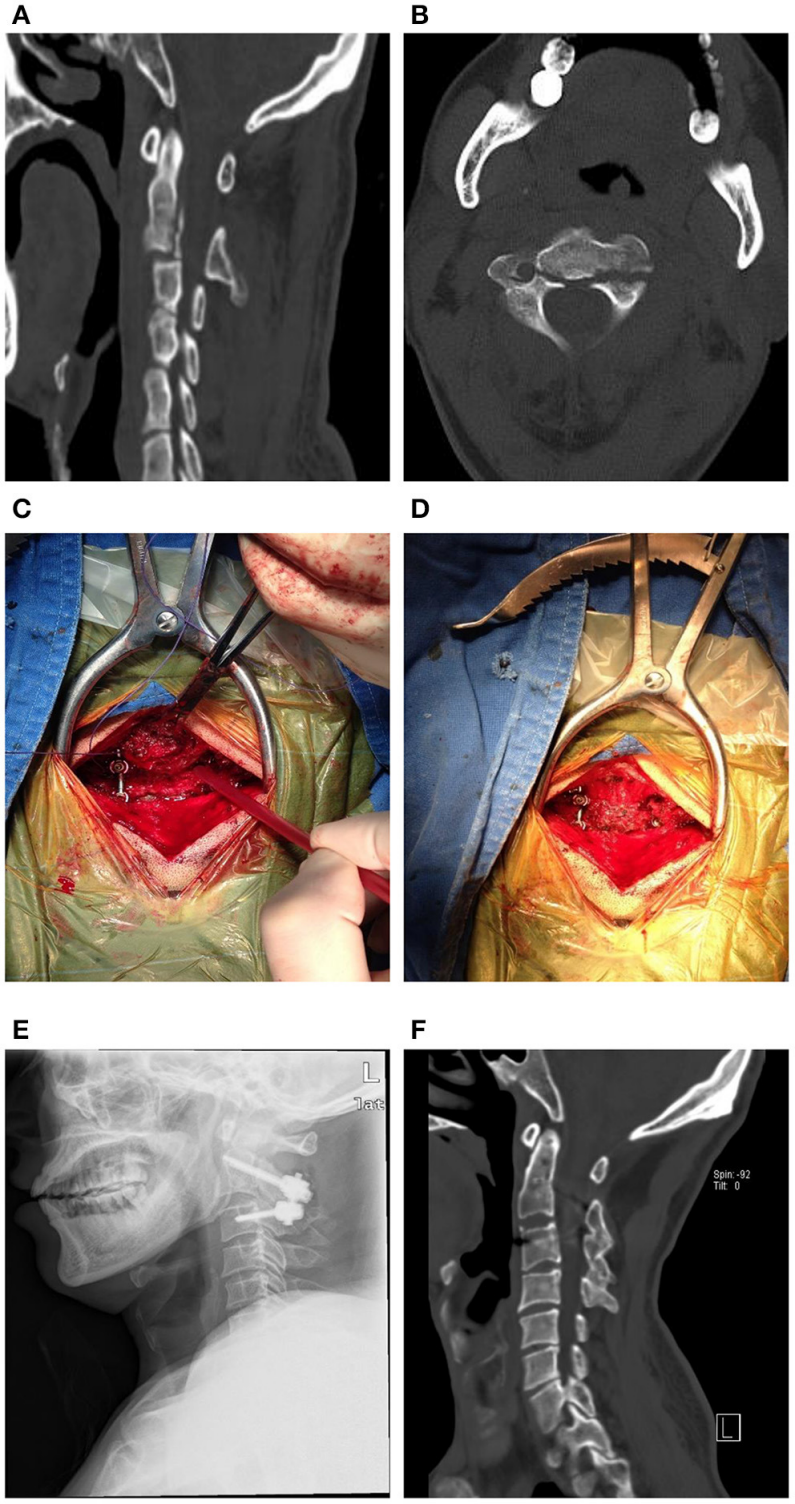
(A,B) Preoperative cervical CT-scan of a 42-year-old male patient with instable Hangman fracture. (C,D) The screws were inserted in C2-3 pedicle and C2 spinous muscle complex was displaced into C2-3 posterior arch. (E) The postoperative X-ray showed good reduction and realignment. (F) The posterior cervical CT-scan at 1-year follow-up visit showed the bone fusion.
